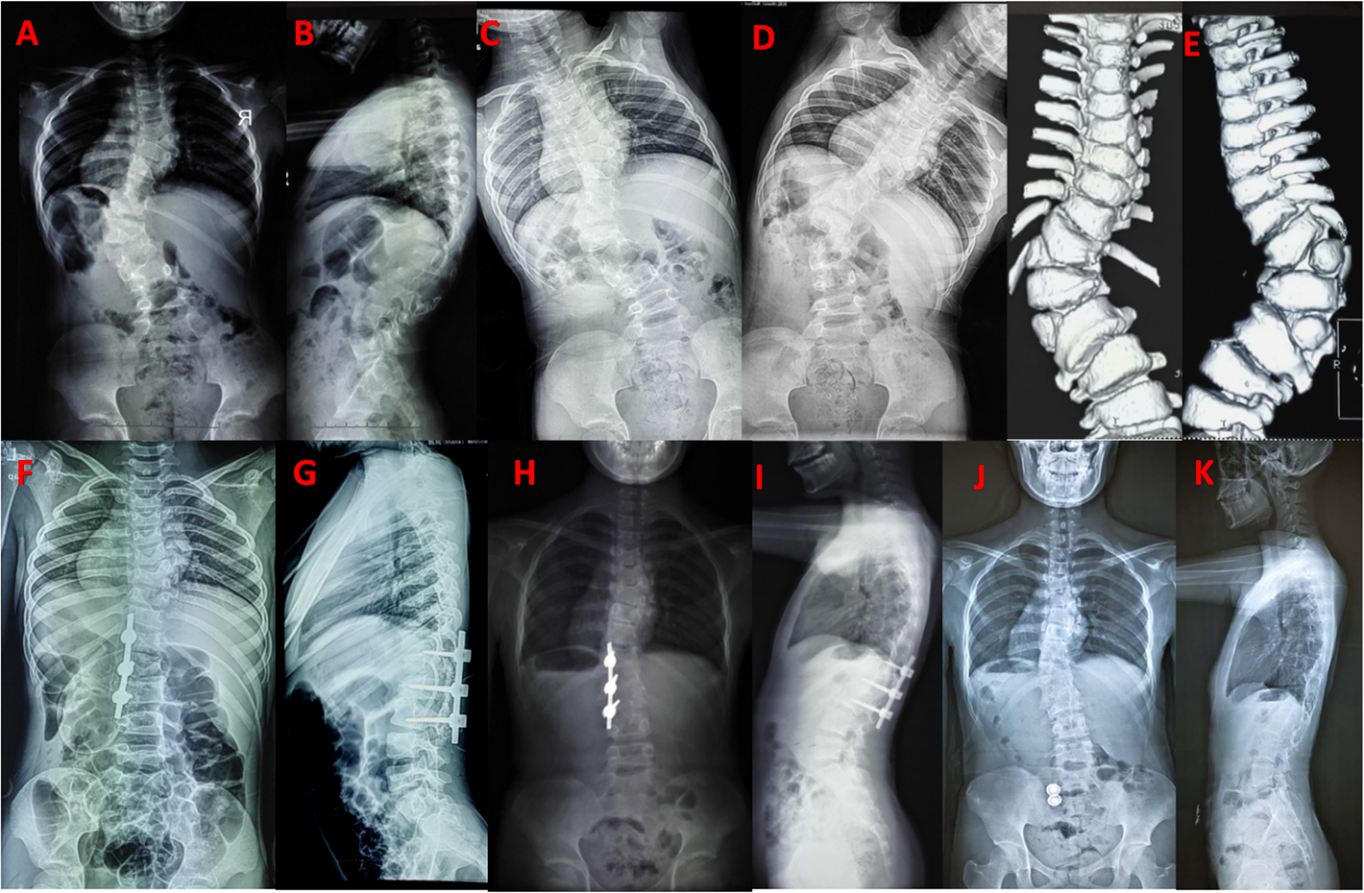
A 8 years old girl with kyphoscoliosis due to T6, 11 and L1 HV. Radiographs images and CT images were obtained preoperatively, which shows T11 and L1 full segmentation HV caused kyphoscoliosis (a, b, e), less flexibility and very rigid curve (c,d). Postoperative PA and lateral radiograph, she was treated with posterior L11, L1 HV resection with unilateral fusion (f,g). PA and lateral radiograph at 46 months follow-up show that correction and trunk balance are well maintained (h,i). Implants removal was required in 6 years after surgery. Final PA and lateral radiograph at 84 months follow-up show that residual curve and trunk balance are stable (j,k)
